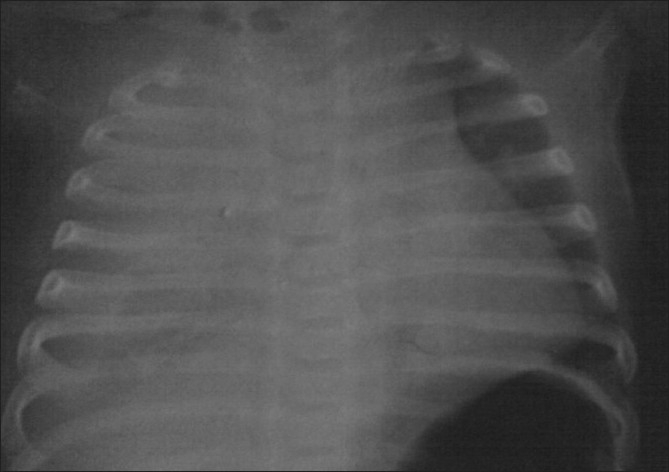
Chest radiograph showing unilateral opacity of right hemithorax and shifting of airway and heart on left side
